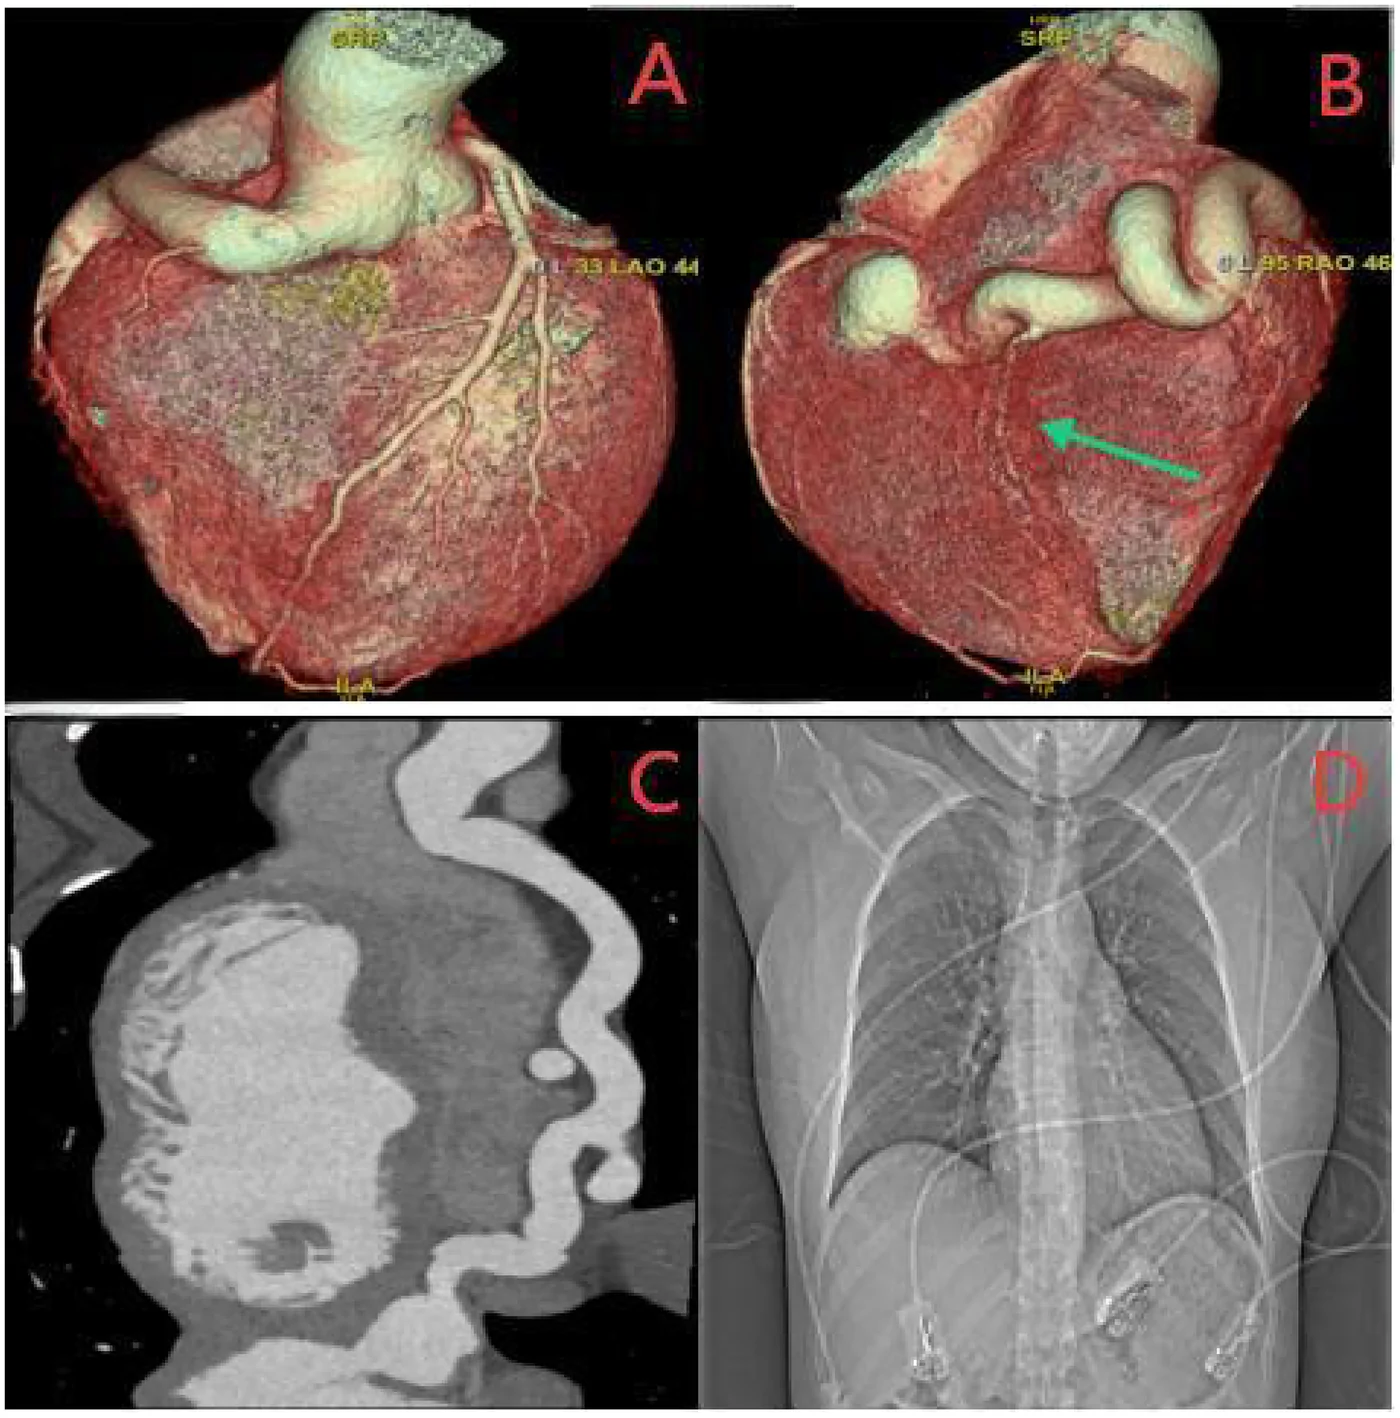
(A—C) Right Coronary artery-to-left ventricular Fistula. Green arrow: PDA in relation to the coronary artery fistula. (D) Chest radiograph was unremarkable.
